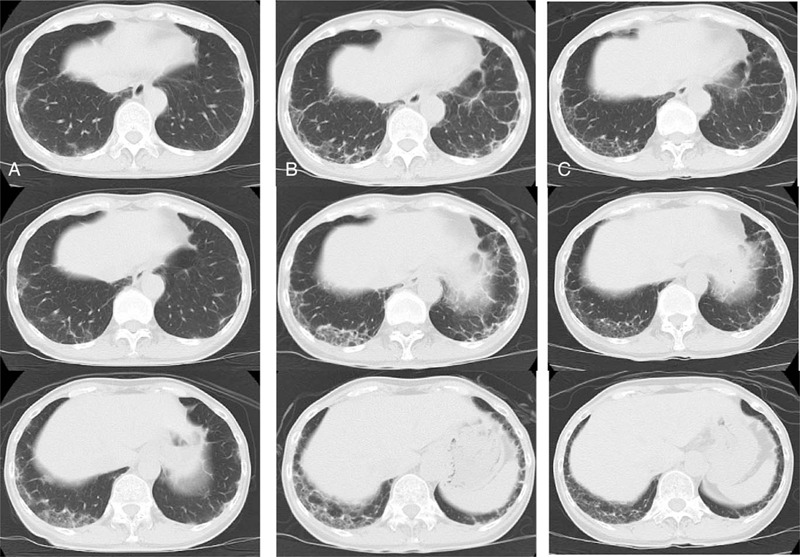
Chest computed tomography. (A) Chest computed tomography (CT) findings on July 2018. (B) Chest CT findings before the recommencement of prednisolone and introduce of tofacitinib (on November 2018). (C) Chest CT findings 6 months after tofacitinib introduction (on May 2019).
